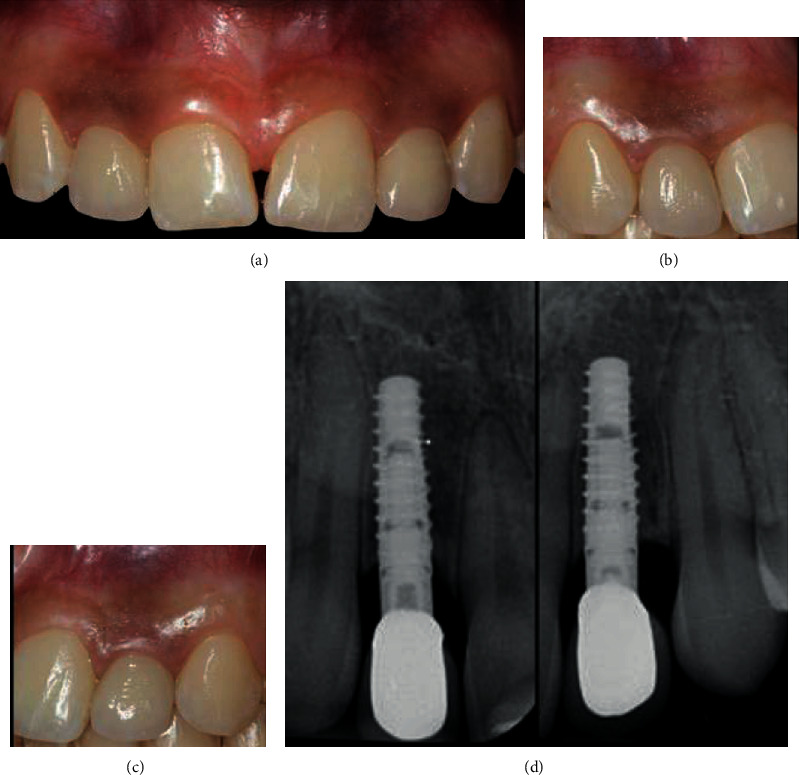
(a) Facial view of the definitive restorations at the 24-month follow-up. (b) Right lateral view of the definitive restorations at the 24-month follow-up. (c) Left lateral view of the definitive restorations at the 24-month follow-up. (d) Periapical radiographs at the 24-month follow-up.
